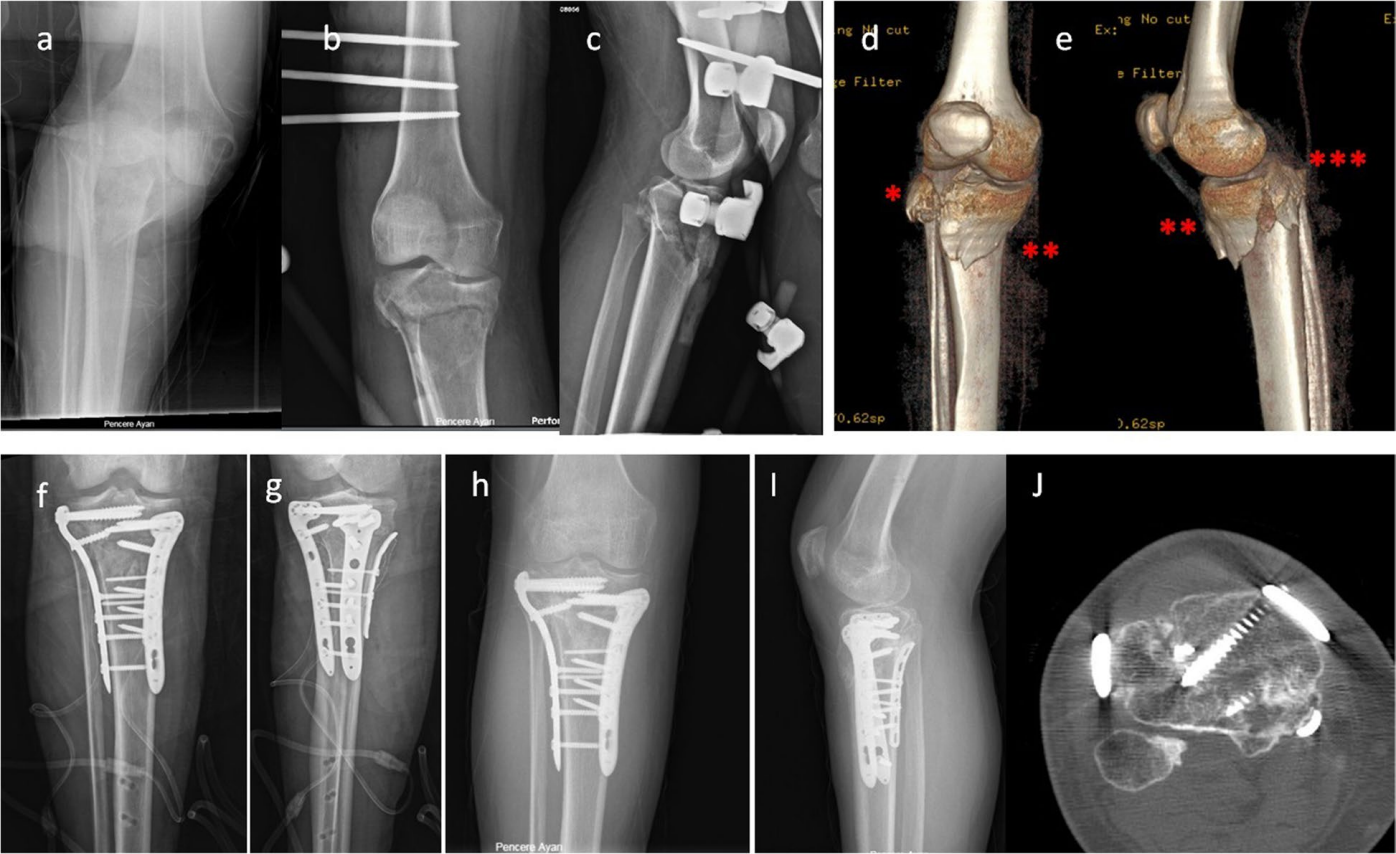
a–c A patient sustaining right plateau fracture after a traffic accident. Provisional reduction was obtained with provisional unilateral external fixators. d, e 3D CT revealed fractures of three columns. *Lateral column, medial column, *posterior column. f, g The patient underwent definitive surgery on day 21 after trauma. The patient was treated using two incisions for fixation of three columns with three plates. Anatomical reduction was obtained during surgery. h–j X-ray and CT at 16 months postoperatively showed perfect union of the fracture. j Axial CT showing fixation and union of three individual columns
